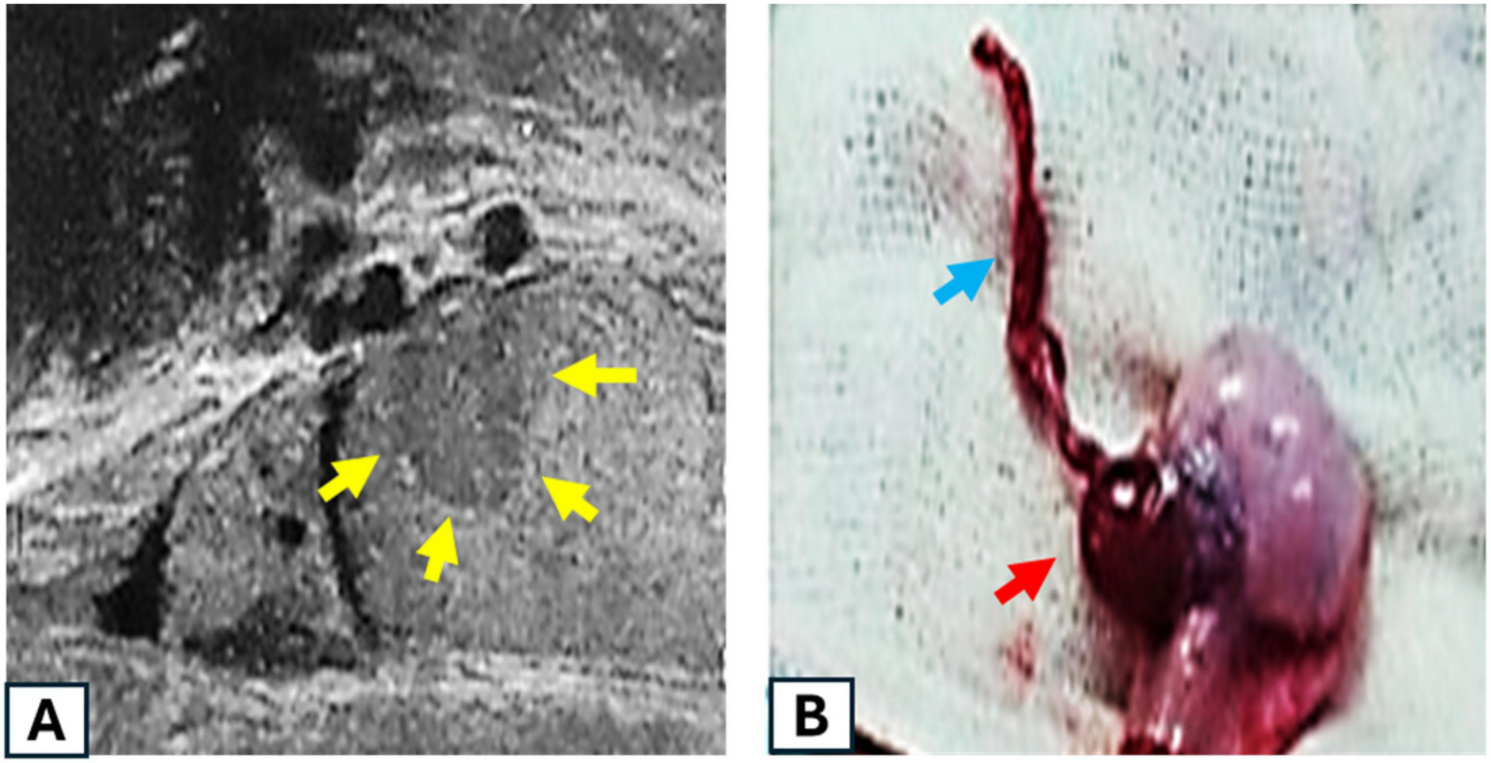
(A) First case: scrotal ultrasound revealing a hypervascular, hypoechoic testicular nodule measuring 4 mm × 6 mm × 5 mm (indicated by yellow arrows). (B) Second case: intraoperative images showing a mass attached to the head of the epididymis (red arrow) and proximally connected to a cord-like structure (blue arrow).
